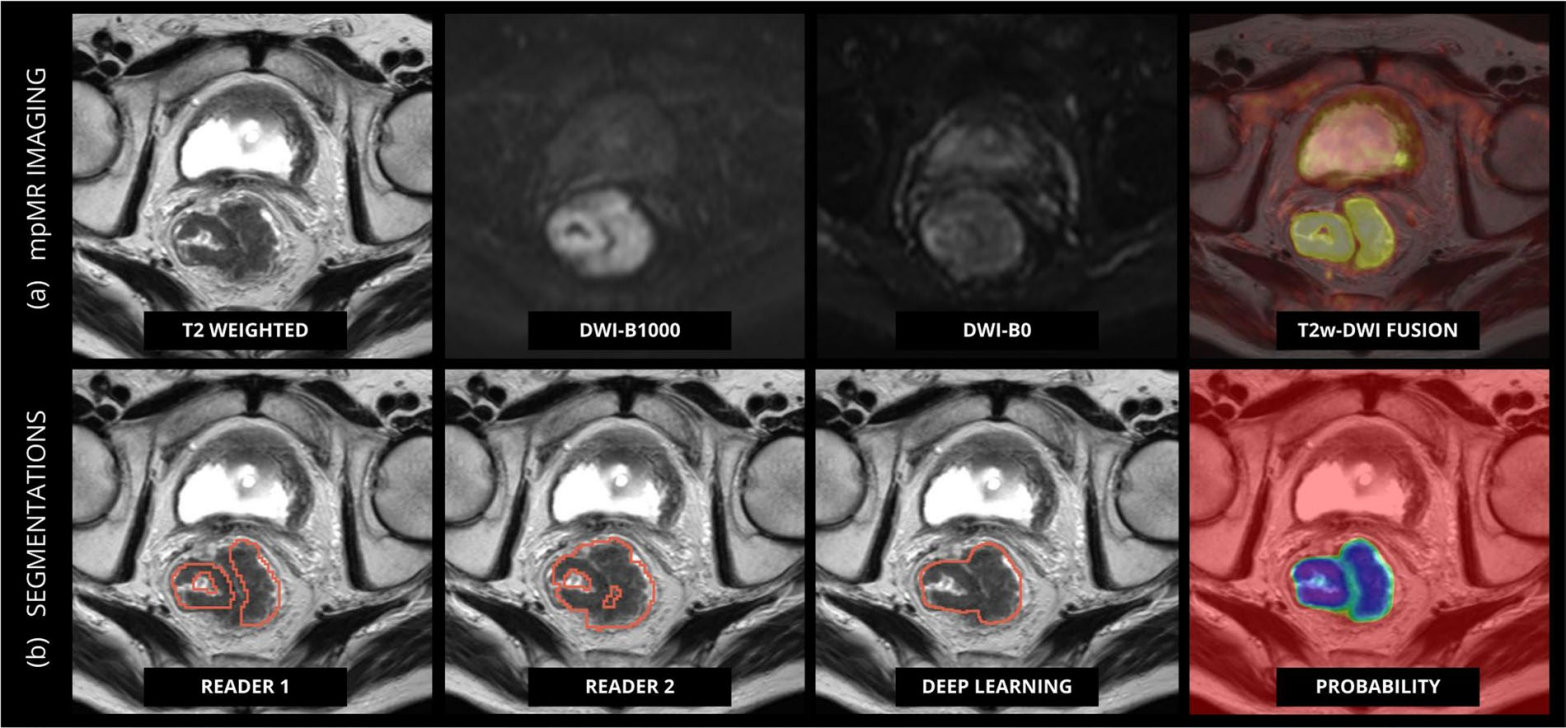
Example of Multiparametric MR in a rectal cancer patient. mpMR of the pelvis of a male patient with rectal cancer before the start of the treatment. Corresponding slices of different sequences on the transversal plane are shown. (a) The sequences are, in order: T2 weighted, DWI B1000, DWI B0 and fusion imaging between T2 weighted and the DWI B1000. Notice how anatomical structures and tissues surrounding the tumour – such as prostate, bladder, and seminal vesicles – and artefacts in general show the same hyper-intensity on the DWI of the tumour. (b) Delineations of the tumour done by (from the right left hand side): the experienced reader used for the training, the independent reader, the result of the algorithm and the corresponding probability map generated by the algorithm.
